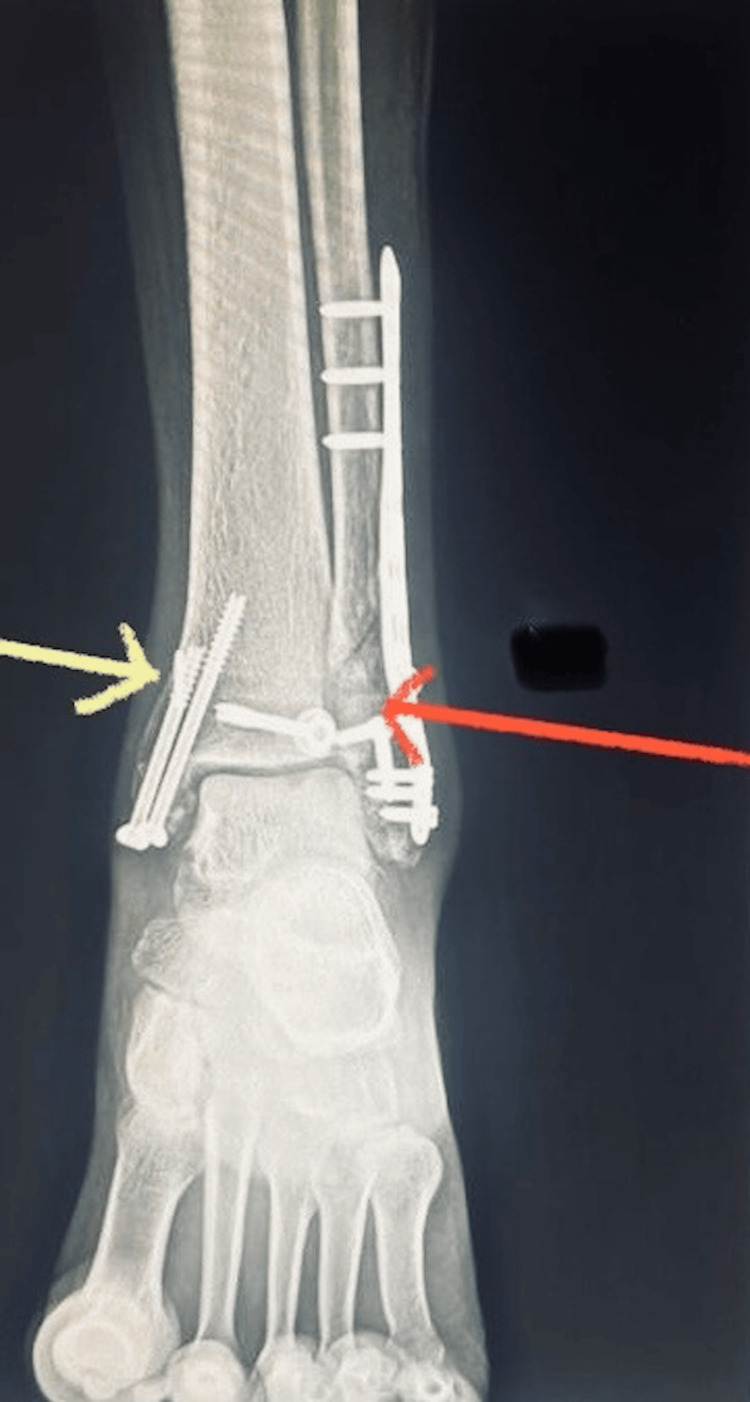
Post-operative X-ray (anteroposterior view) of the left ankle. Yellow arrow indicates the metal button medial distal tibial cortex and red arrow indicates the transverse lucent tunnel used for Arthrex TightRopes.
